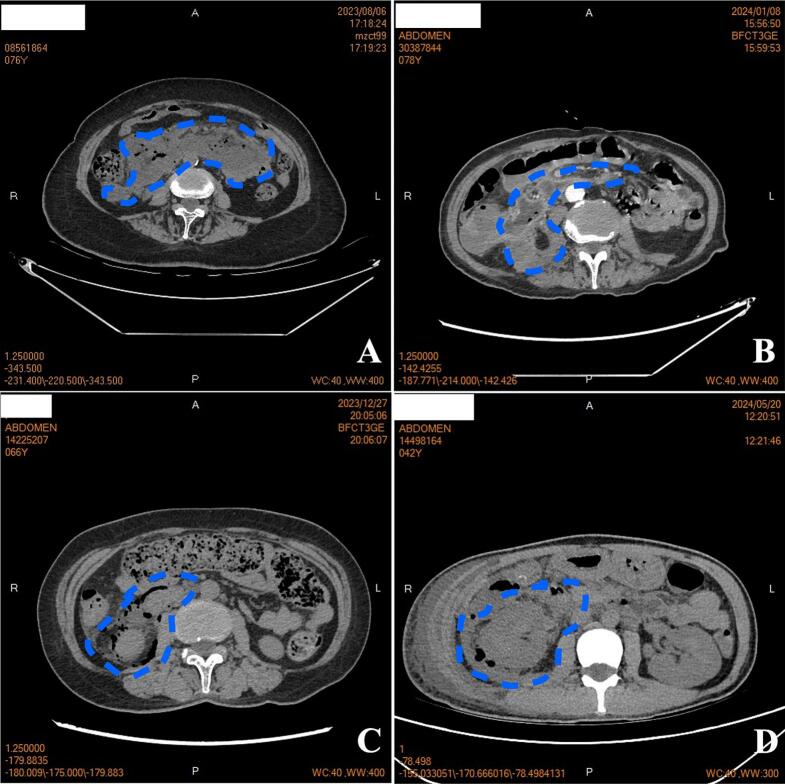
CT findings at initial diagnosis of retroperitoneal duodenal perforation. A, B, C, D were for case 1, 2, 3, 4 respectively. The area encircled by the blue line represents the exudate or gas accumulation in the retroperitoneal space.
